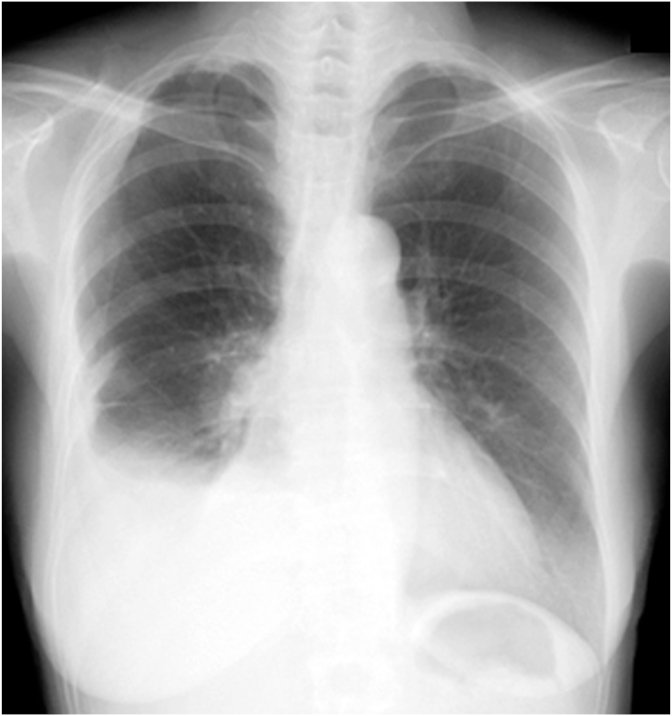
Chest radiographs taken at in April 2008. Masses were observed in the area corresponding to the peripheries of the right lower lung field and the right lateral chest wall.
